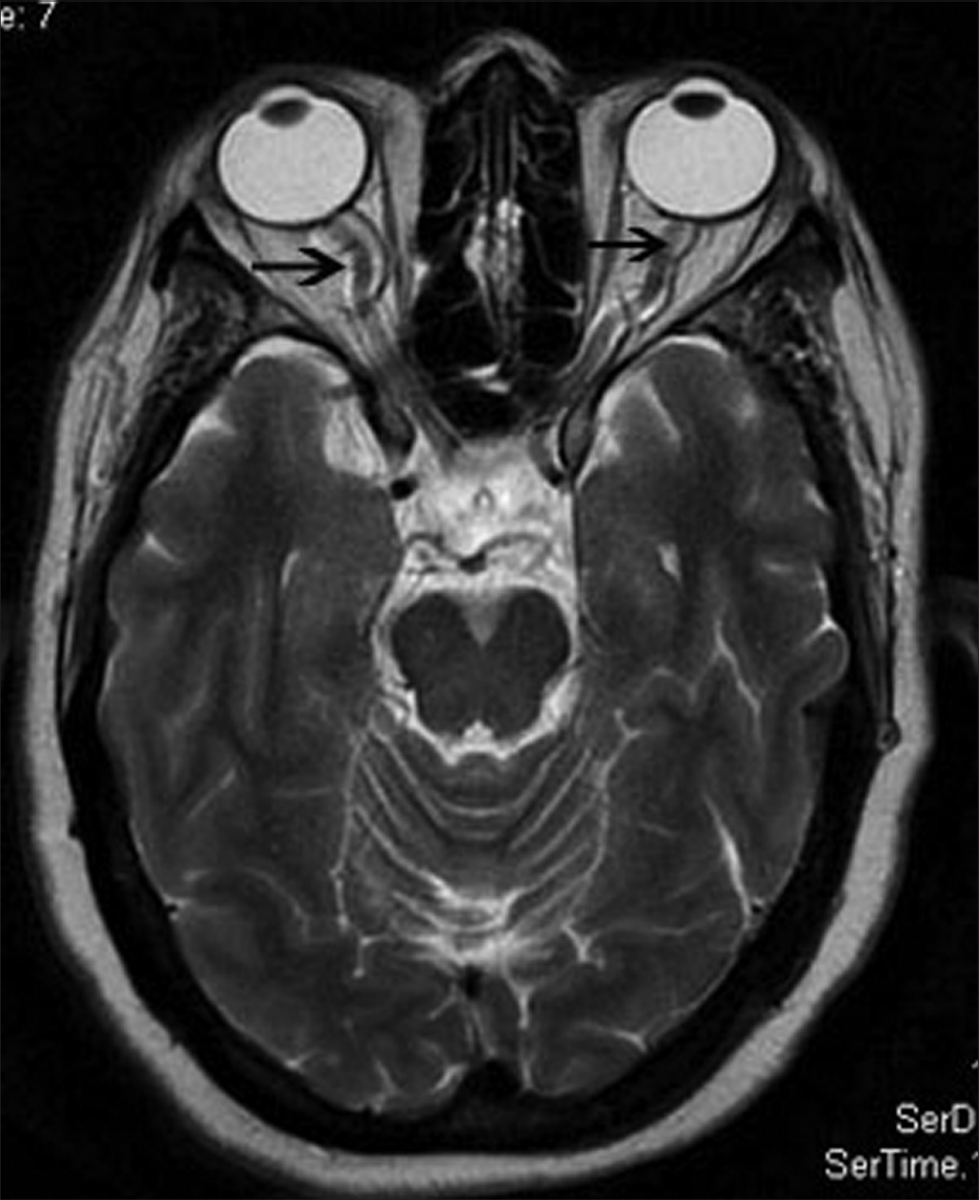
Transverse T2-weighted image shows vertical tortuosity and elongation of the optic nerve (black arrow)
